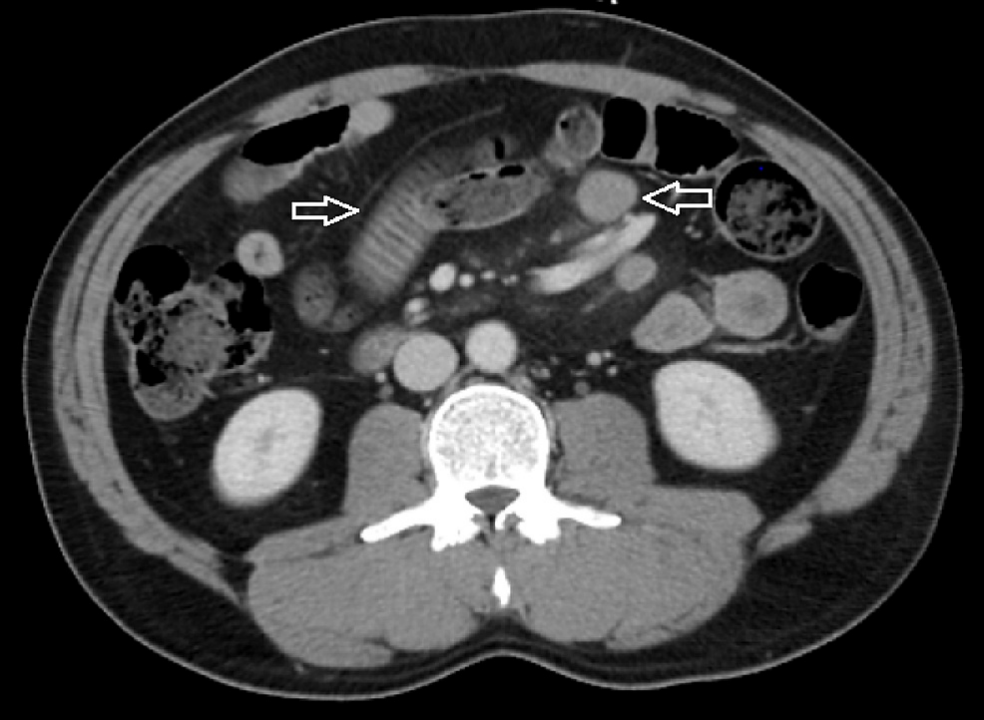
CT of the abdomen and pelvis showing multiple fecalized loops of small bowel without definitive transition point concerning for early/partial small bowel obstruction. There is mesenteric lymphadenopathy (measuring up to 1.9 cm) (right arrow) in conjunction with mural thickening of the small bowel along with slight aneurysmal dilatation of the lumen concerning for small bowel lymphoma (left arrow).
